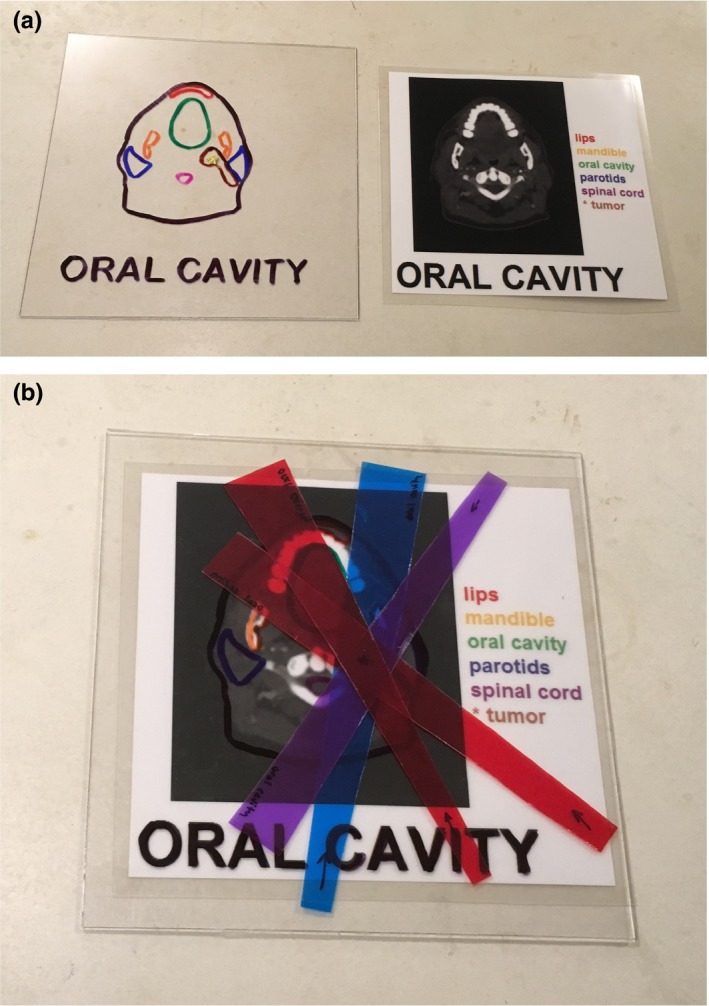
Materials used for the treatment planning activity. In (a), the oral cavity case is shown with the acrylic sheet (left) traced with color‐coded anatomical structures. The isocenter is marked with a gold star. The corresponding printed copy (right) of anonymized patient computed tomography data includes a list of target and avoidance anatomical structures, with the target structure marked with an asterisk. In (b), an example of a possible beam placement geometry is demonstrated, with transparency beams overlapping over the target region and healthy tissue avoided where possible.
